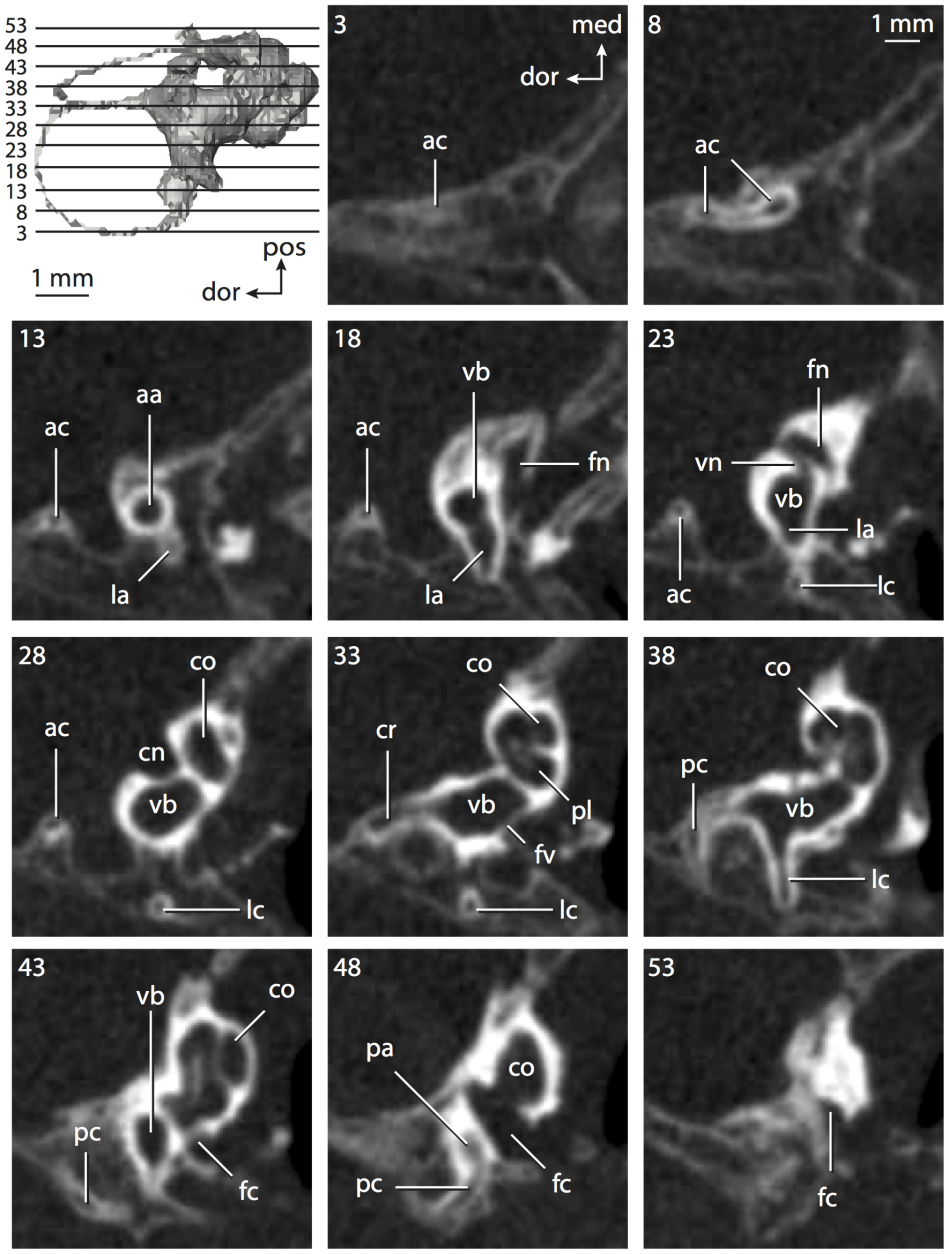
CT slices through ear region of*Atelerix albiventris*. Abbreviations listed at the end of the Materials and Methods section.
